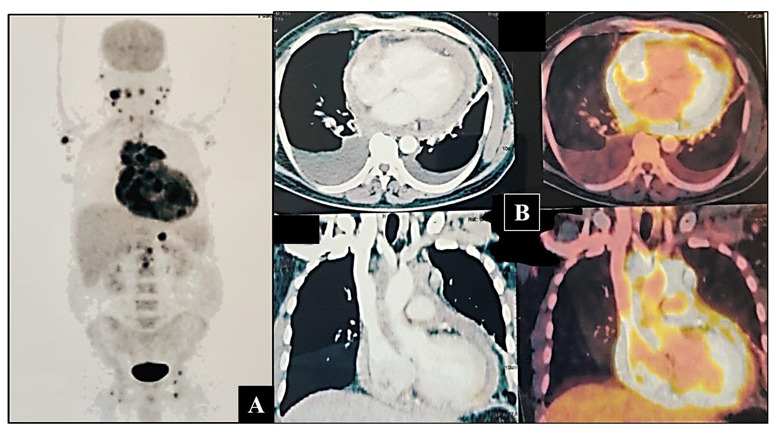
(A) Maximum intensity projection (MIP) image of 18FDG PET-CT scan showing increased uptake of FDG around the cardia, neck, axillary, and inguinal nodes. (B) Axial and coronal sections showing increased uptake by the lesion, surrounding cardia, and great vessels.
